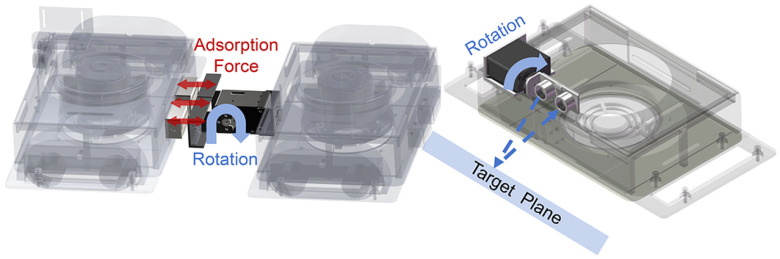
Detection and transition mechanism. As the servo motor rotates, the ultrasonic sensor measures the distance, which is then used to calculate the angle. The electromagnets can generate an attraction force of 200 N in attachment direction. After the two modules are attached electromagnetically, the servo motor rotates to complete the wall transition.
